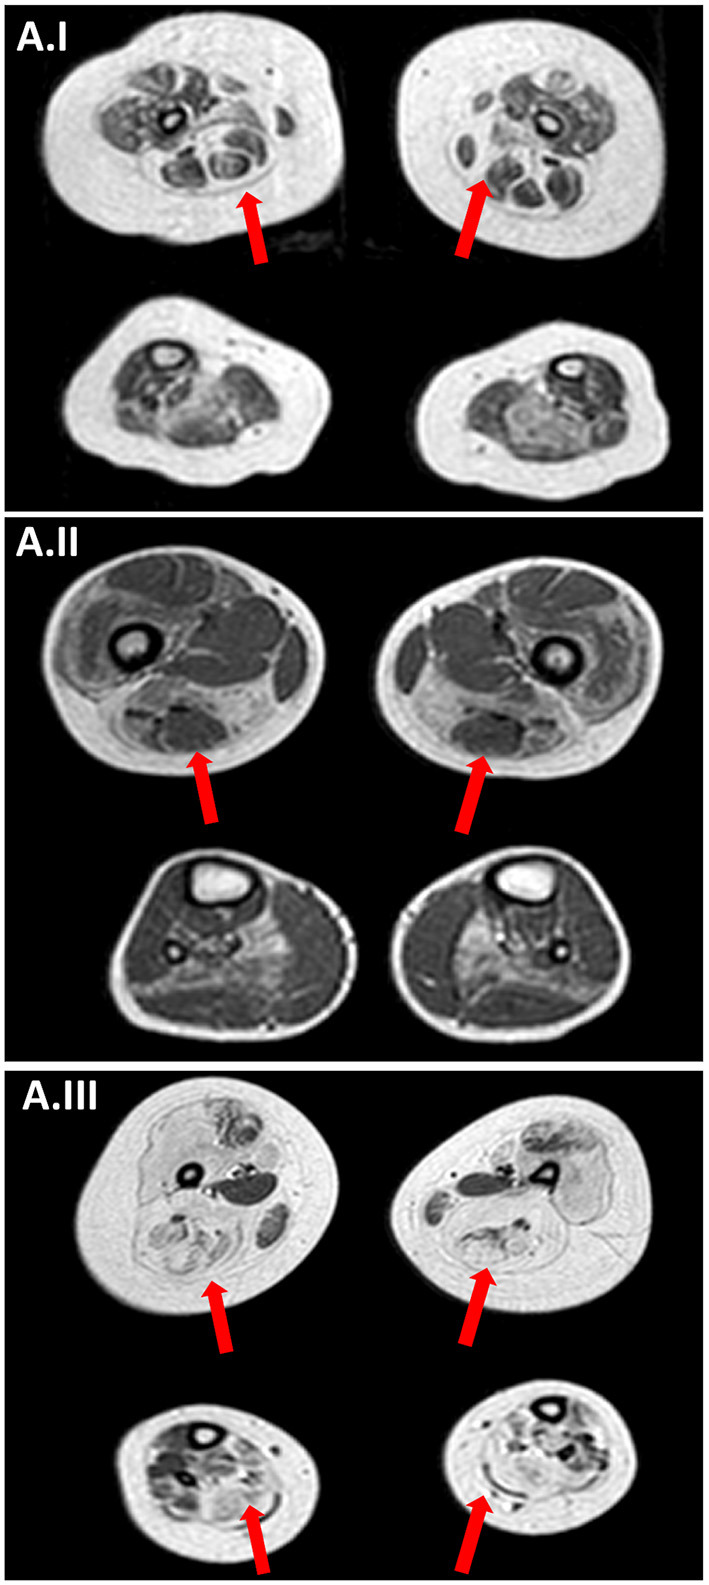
Muscle MRI. (A.I) Muscle MRI (Patient II; 18-months old) axial TSE T1 images at thigh and lower leg level showing a bilateral and symmetrical atrophy and fatty infiltration of gluteus maximus, sartorius, adductor magnum and, at lower leg level, in soleus muscle a pattern compatible with *RYR1*-RCM. (A.II) Muscle MRI (Patient III.1; 5 years old) axial TSE T1 images at thigh and lower leg level showing a bilateral and symmetrical atrophy and fatty infiltration of vastus lateralis, sartorius, adductor magnum and, at lower leg level, in soleus muscle a pattern compatible with *RYR1*-RCM. (A.III) Muscle MRI (Patient III.2; 7 years old) axial TSE T1 images showing bilaterally muscle atrophy with diffuse fat substitution of pelvic girdle muscles thigh and lower leg muscles, with relative sparing of rectus femoris, adductor longus, gracilis, and tibialis anterioris.
